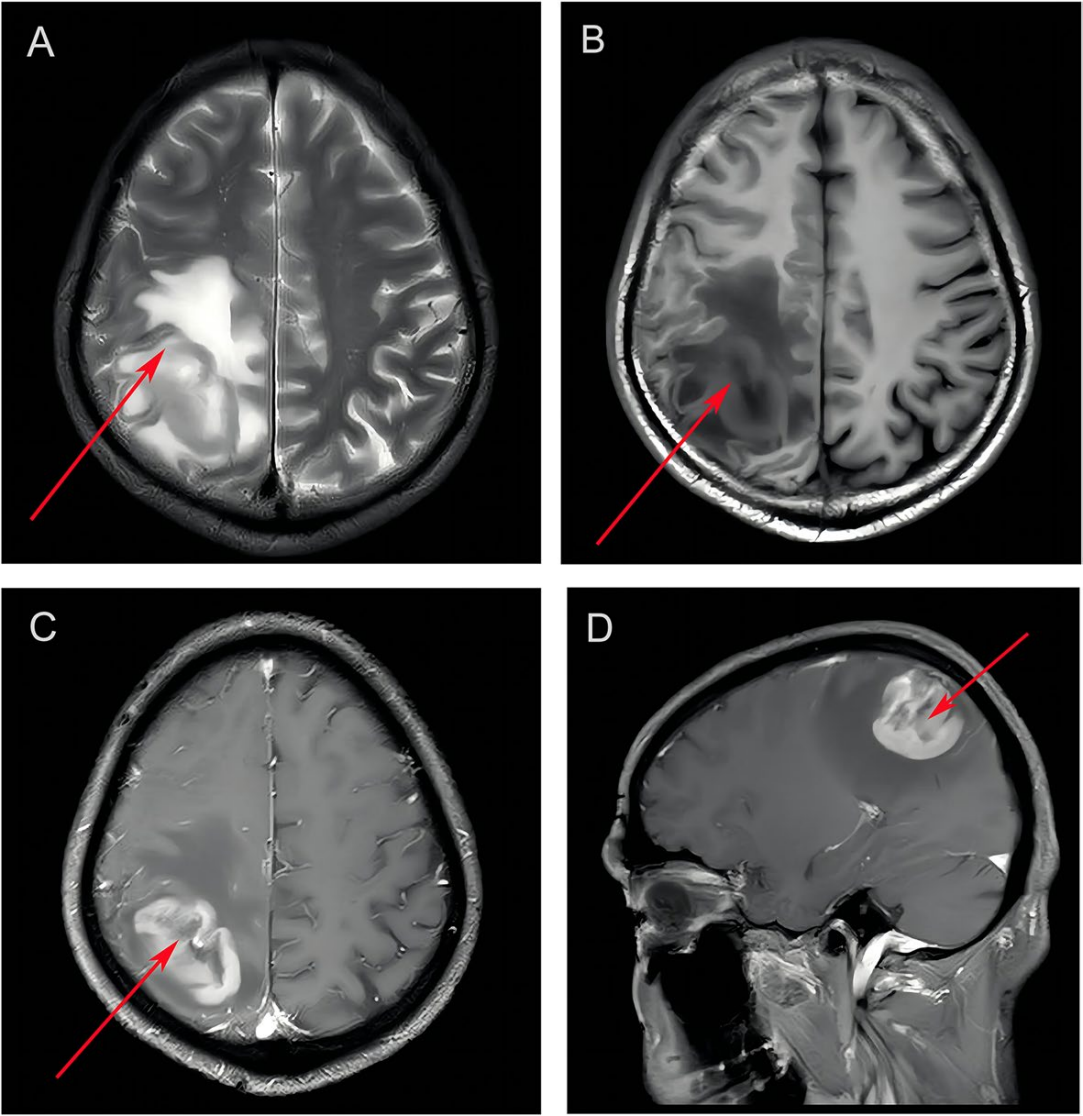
Typical brain MRI imaging results of patients with glioma. A Transverse plane (unenhanced MRI) T2W1. B Transverse plane (unenhanced MRI) T1W1. C Transverse plane (unenhanced MRI) enhanced T1W1. D Midsagittal plane (MRI) enhanced T1W1
